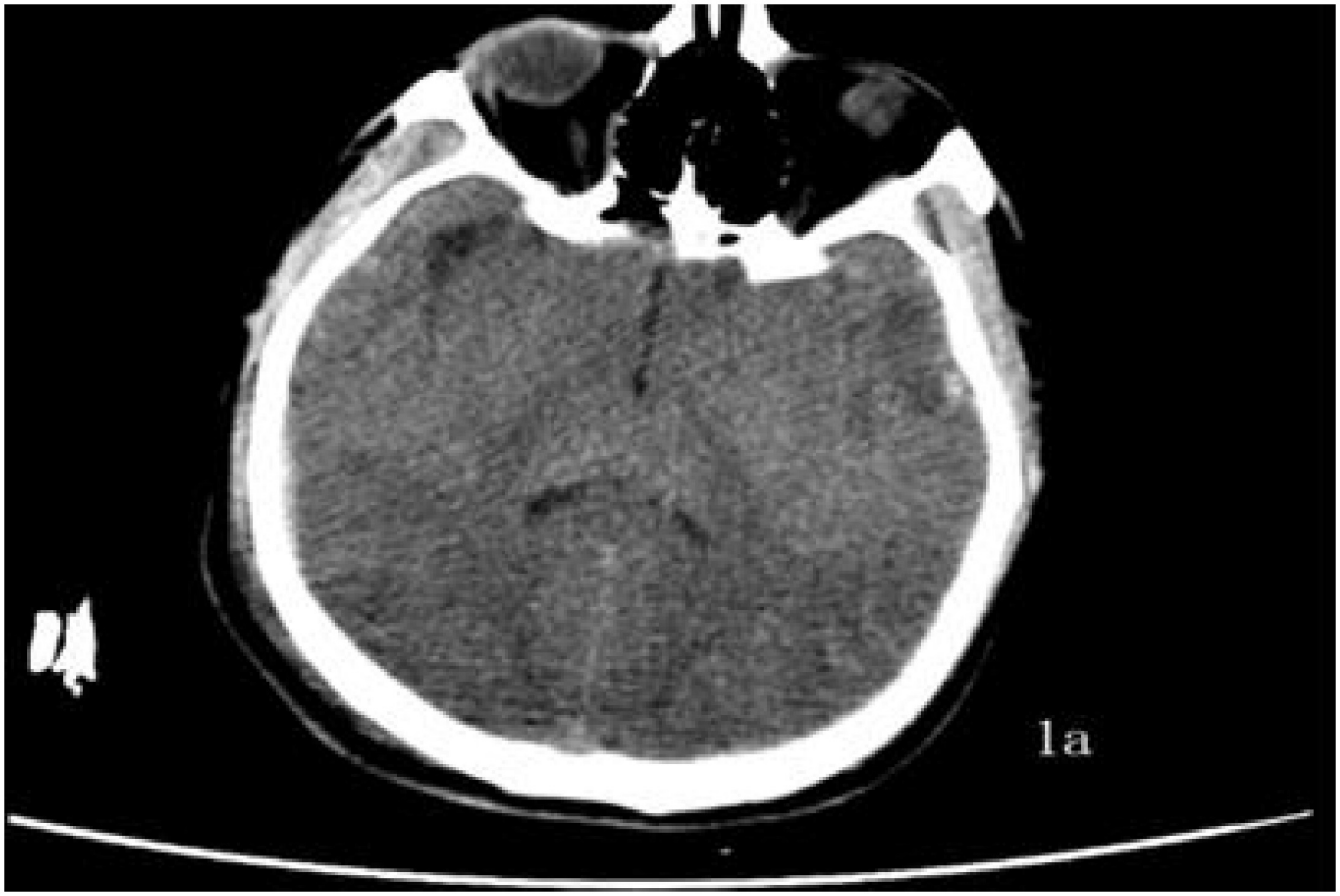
Cranial CT (a): 13 June–cerebral contusion with laceration, subarachnoid hemorrhage, and skull-base fracture visible.
